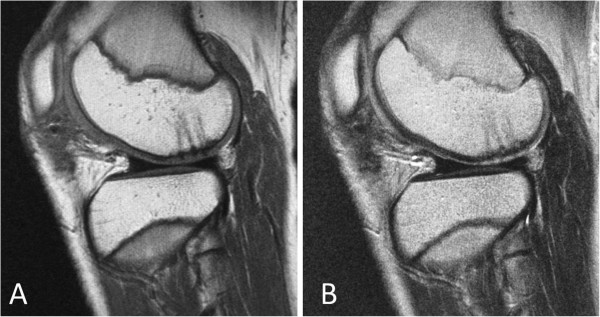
Postoperative magnetic resonance images at 4 months showing apparent healing of the fixed fragment. (A) T1-weighted sagittal image. (B) T2-weighted sagittal image.
